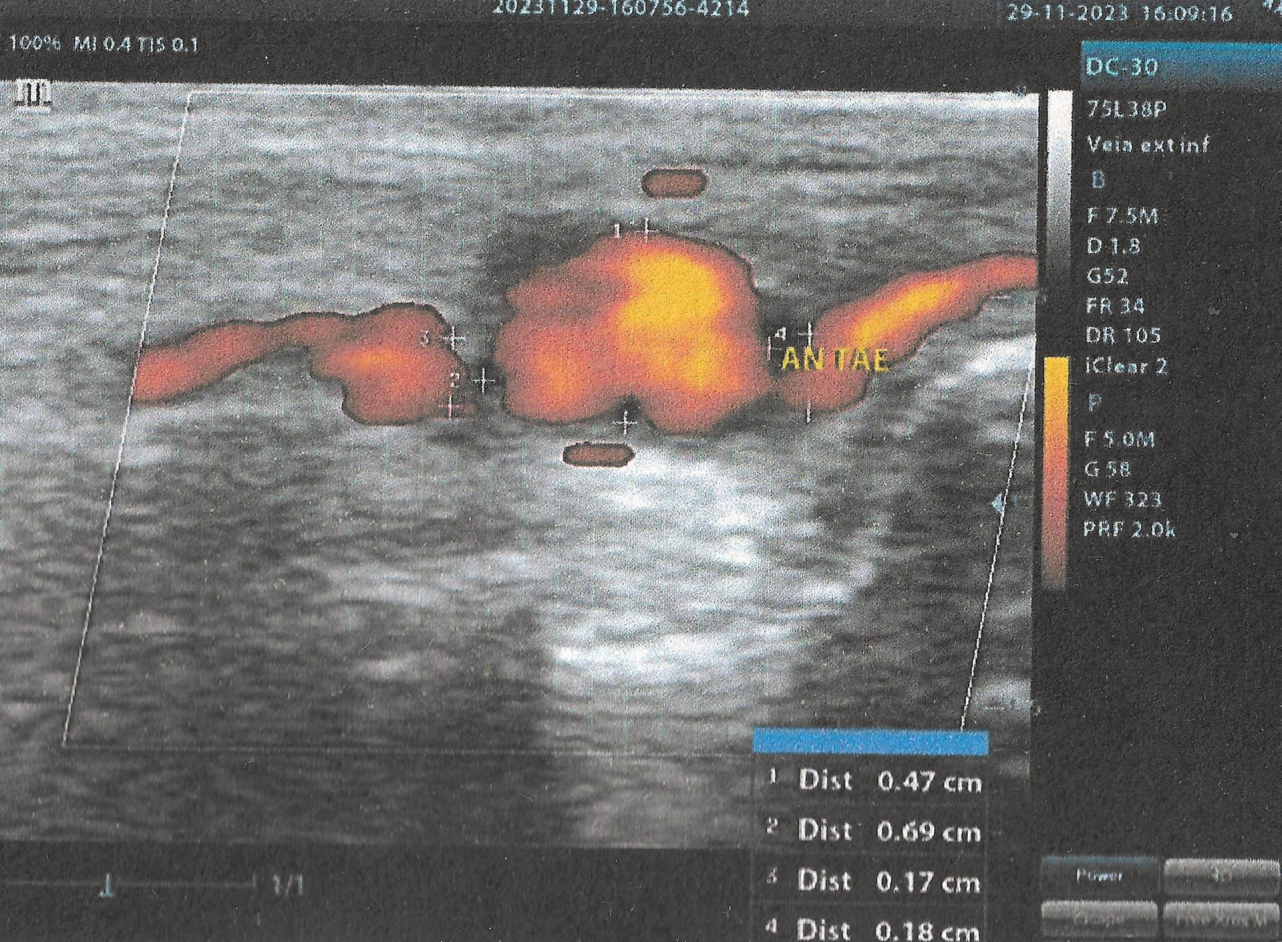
US Doppler scan performed by the vascular surgery team, showing the aneurysm at the transition of the anterior tibial artery to the dorsalis pedis, with turbulent flow in the interior. Size: 0.7 cm side-to-side and 0.5 cm anteroposterior.
